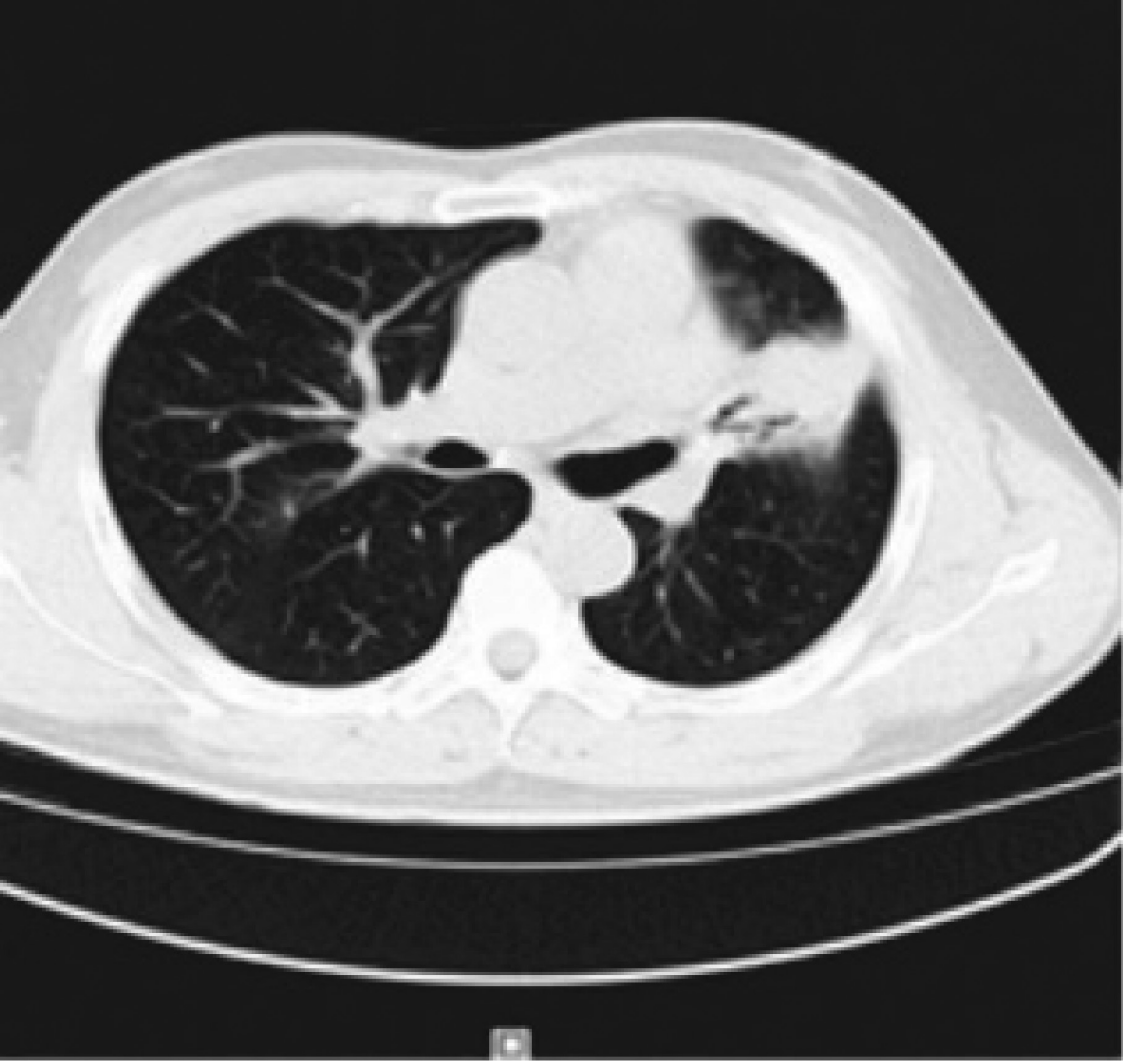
Chest CT after treatment.
